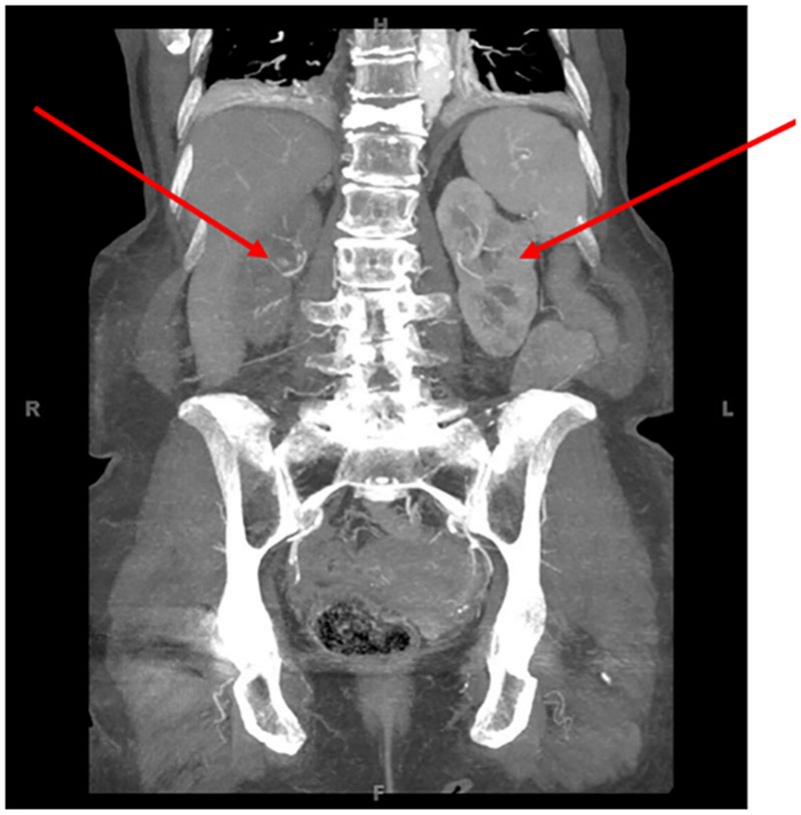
Computed tomography angiogram of the abdomen and pelvis showing a sharp decrease in attenuation of the right kidney due to complete obstruction of the right renal artery.
